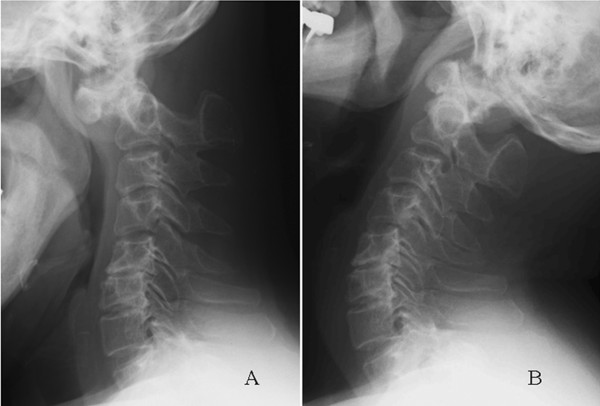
Atlantoaxial instability with absence of the posterior arch of the atlas. Lateral radiographs of the cervical spine during (A) flexion and (B) extension show atlantoaxial instability.
